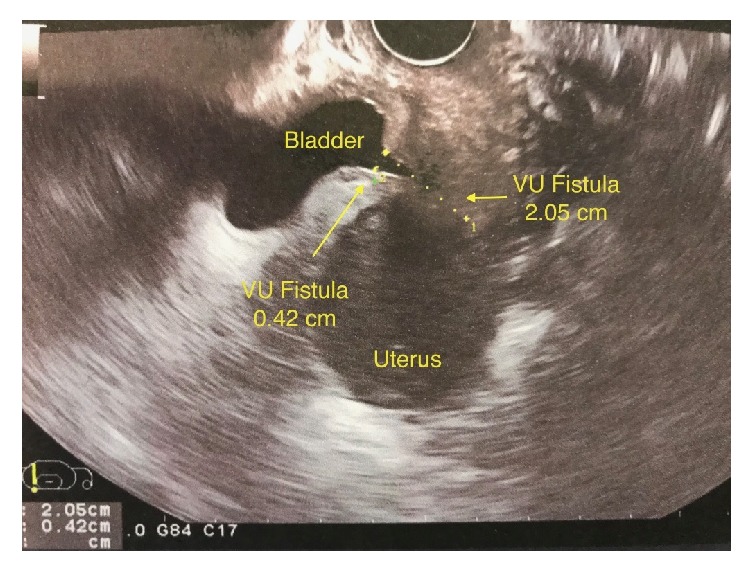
Transvaginal ultrasound revealing the presence of two vesicouterine fistulas.
